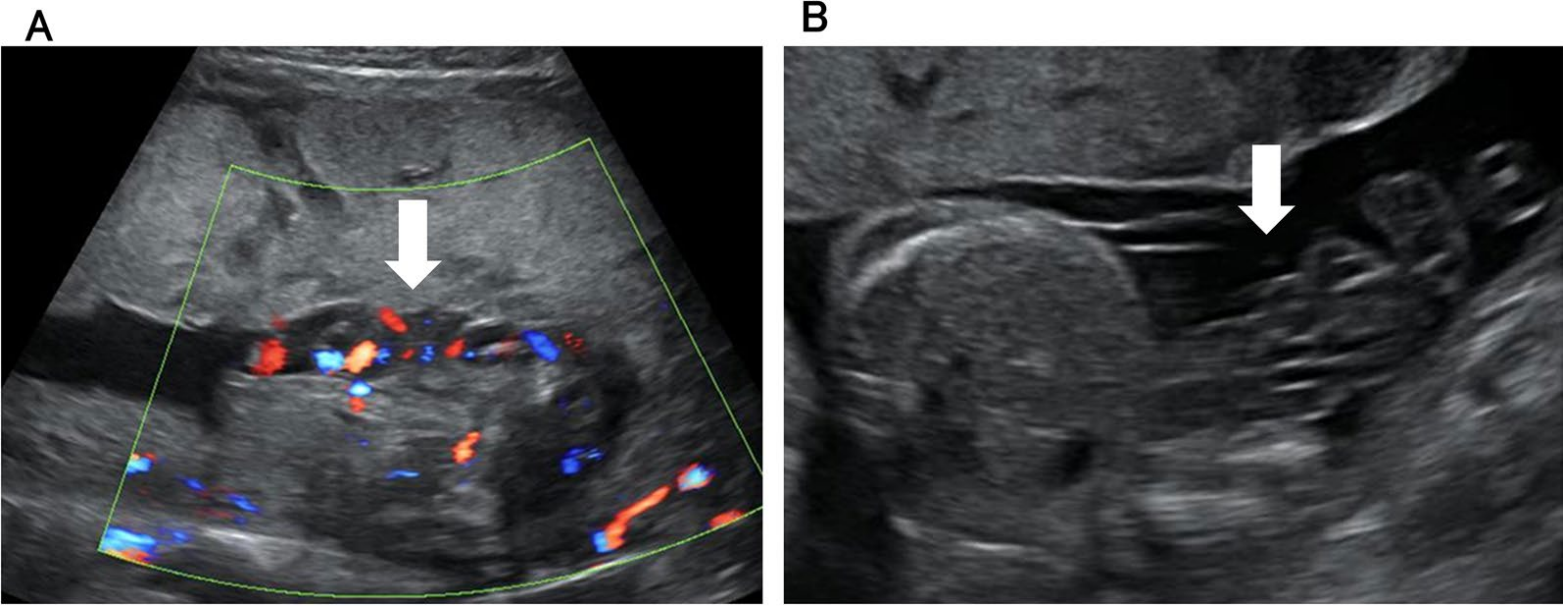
Fetal ultrasonography findings in case 1. A  The “sandwich sign,” that is, the umbilical cord sandwiched between the placenta and the fetus, is observed (white arrow). B The umbilical cord compression has resolved (white arrow)
